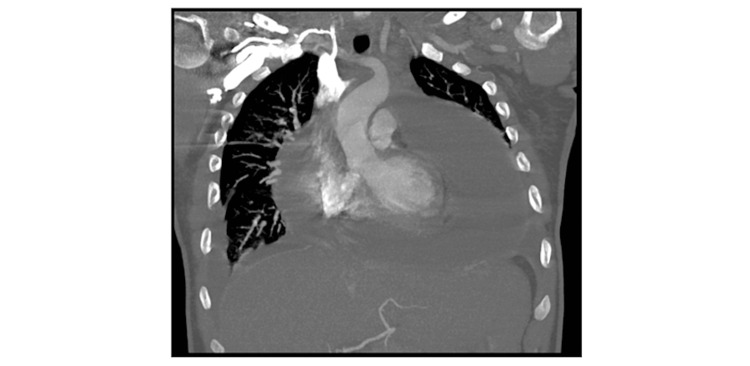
The CT chest shows a large pericardial effusion around the heart.
